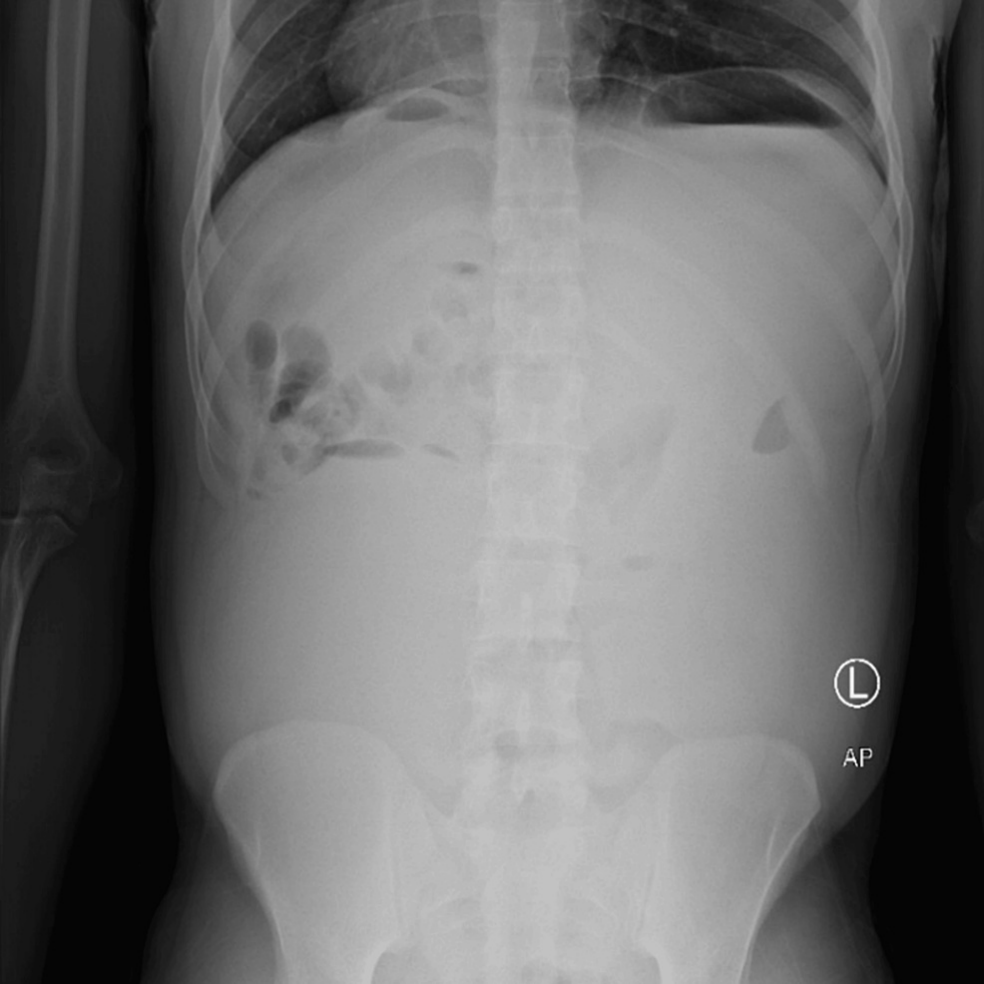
Chest and abdominal x-ray
